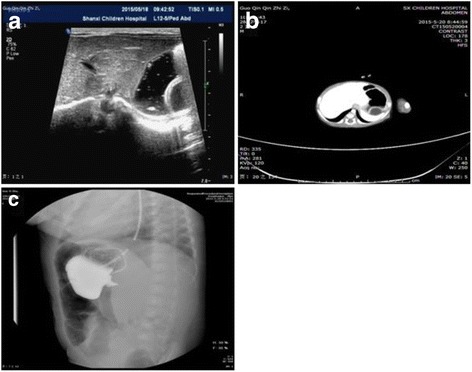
Ultrasound image (a), CT image (b);Pyloric obstruction by radiography (c)
